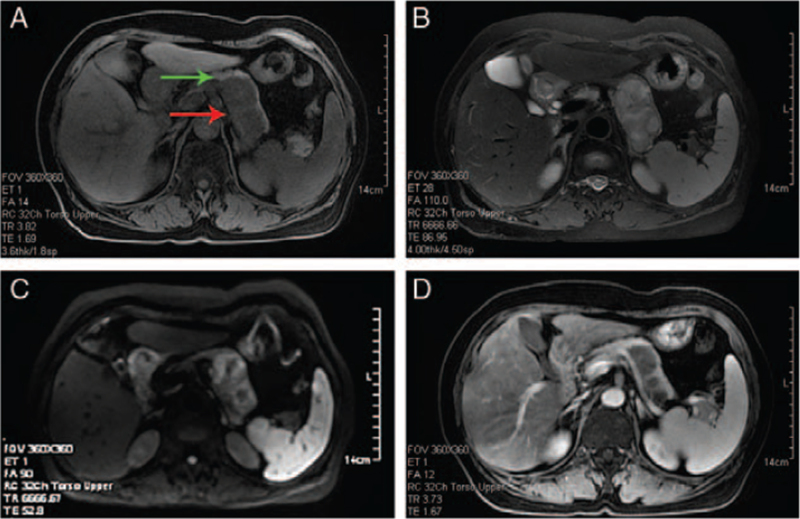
Magnetic resonance imaging (MRI) findings. A: The mass (red arrow) in the pancreas (green arrow) appeared hypointense on T1 weighted images. B: The mass in the pancreatic body appeared inhomogeneous hyperintense on T2 weighted images. C: The tumor appeared inhomogeneous hyperintense on diffusion-weighted imaging. D: On the enhanced MRI, the tumor appeared heterogeneously enhanced.
